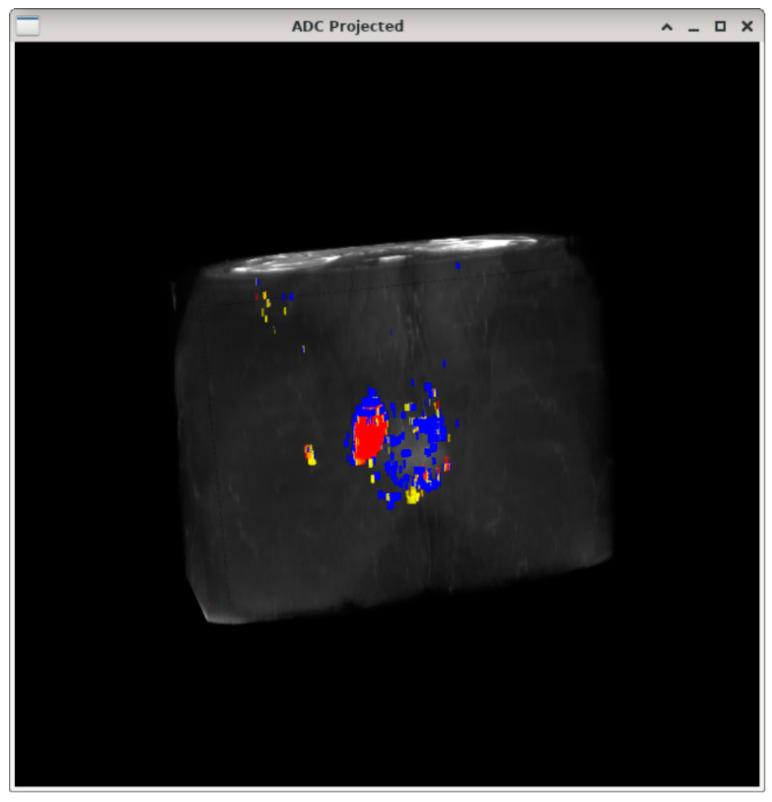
ADC values derived image fused on the T2-weighted sequence. The red region corresponds to the highly cellular tumor area, the blue region corresponds to the low cellular tumor area and the yellow region corresponds to the uncertain tumor area.
